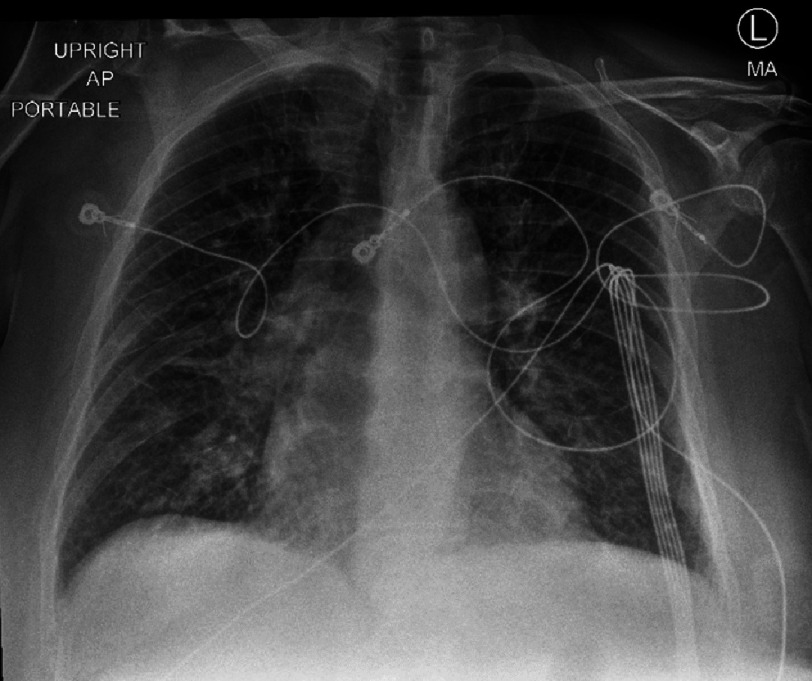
Chest X-ray on admission showing diffuse interstitial opacities.
